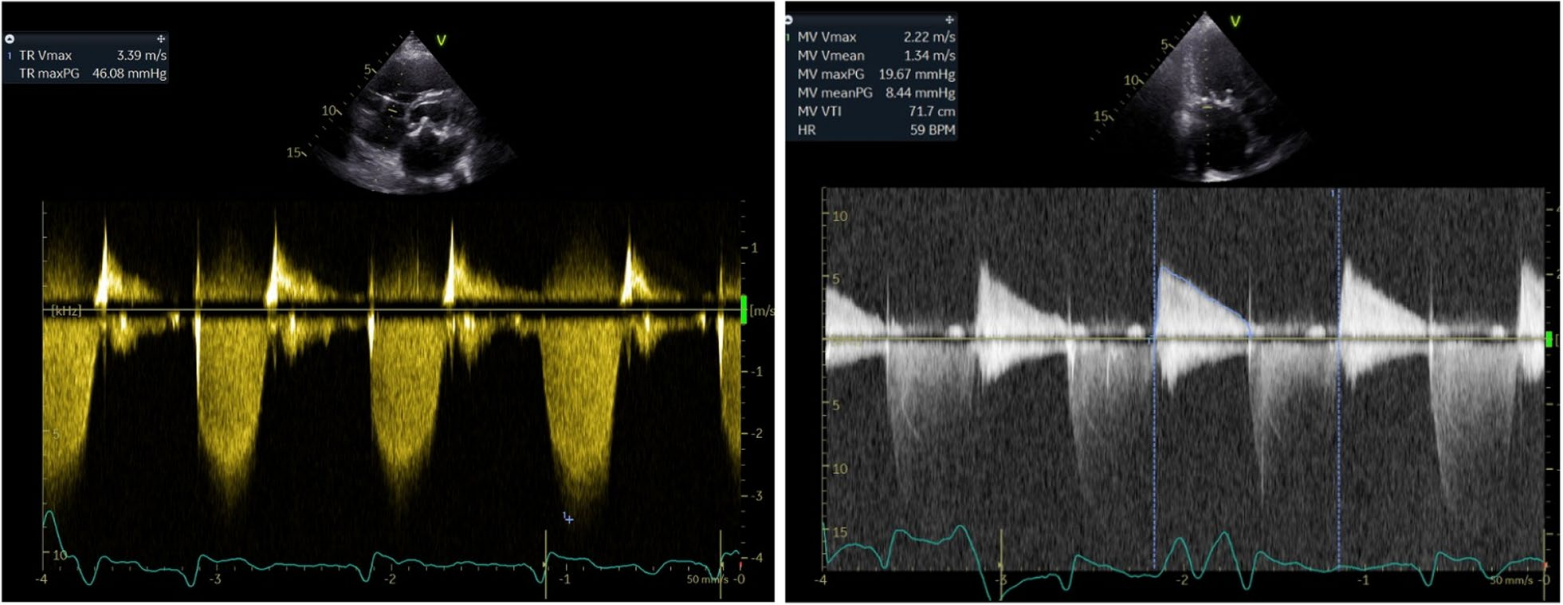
hemodynamic assessment of the valvular lesion with TTE showed mean gradient over the prthesis of 8 mmHg and moderate pulmonary hypertension (45 mmHG1,8, flow + CVP). TTE: transthoracic echocardiography; CVP: central venous pressure
